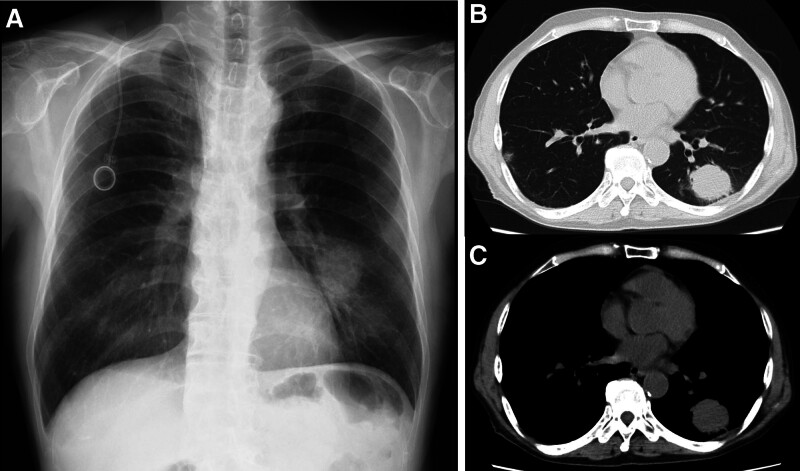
(A) Chest X-ray and (B and C) CT before the initiation of treatment with pembrolizumab: a 40-mm mass shadow in the left lower lobe. CT = computed tomography.
